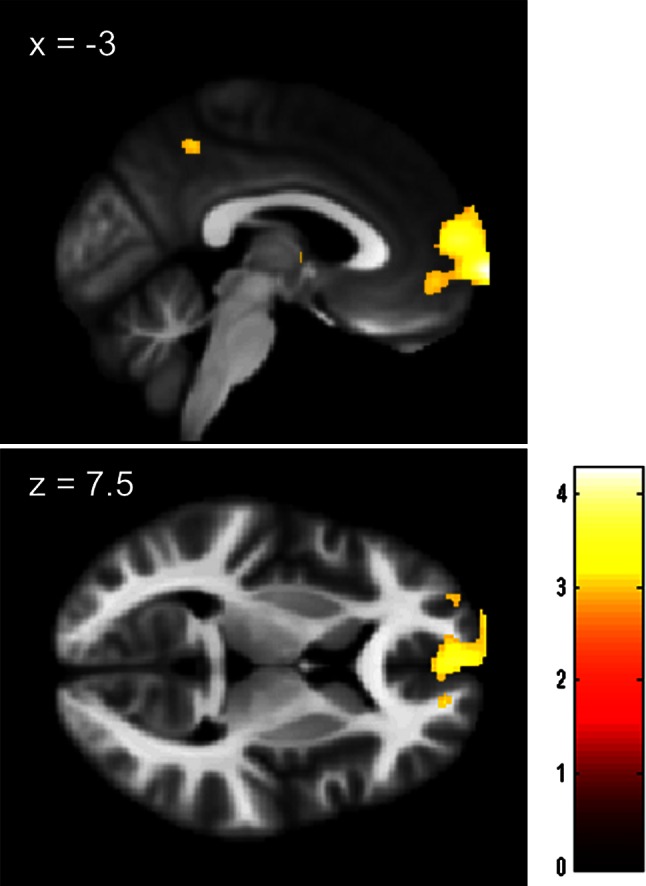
Maps showing larger rGMV in science students than in humanities students. The results are presented at a threshold of *P* < 0.0025, uncorrected, on a skull-stripped image created from the averaged normalized T1-weighted structural images of a portion of the subjects included in this study. The region of significant difference is visible in an anatomical cluster that spreads through mPFC and the frontopolar area. The intensity of the *color* represents the *T* score
